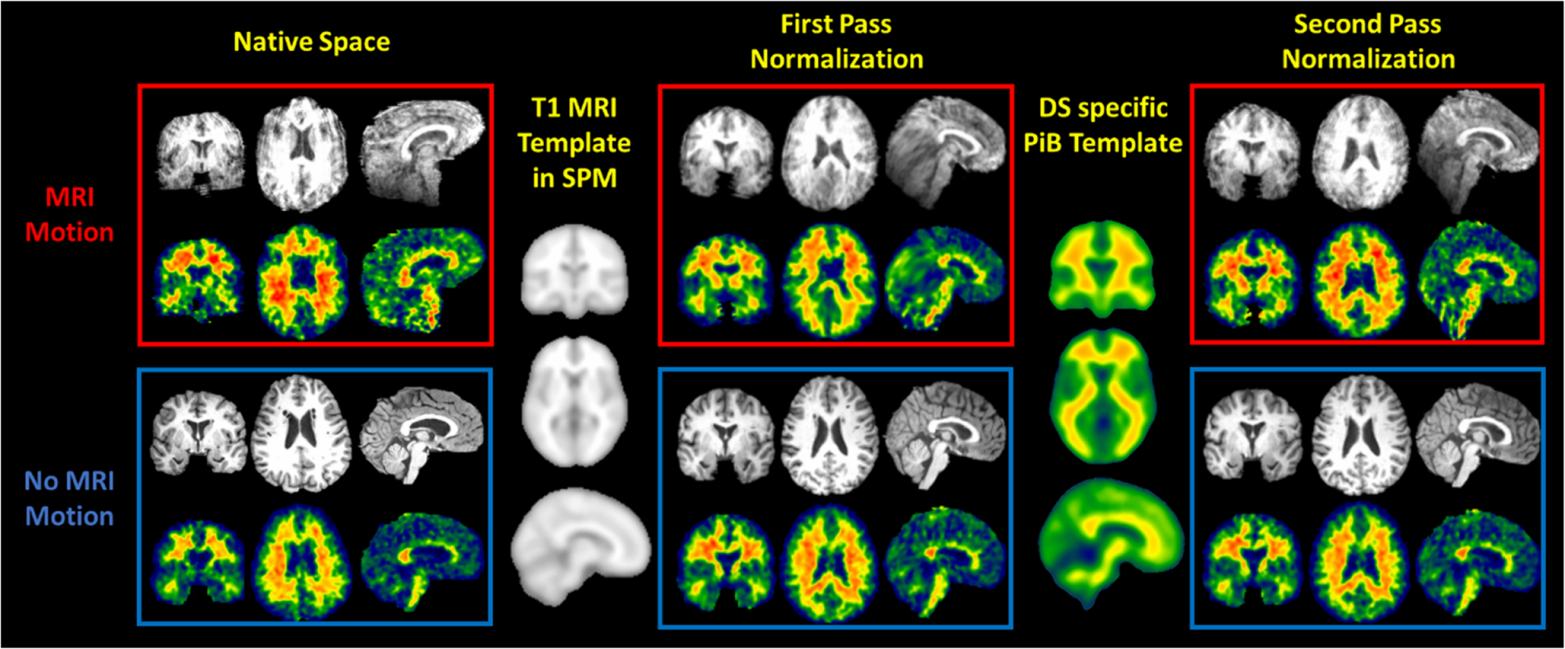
Visual comparison of representative scans with and without MRI motion in native space (red and blue boxes, respectively), and in standardized space after first pass normalization (Native space MRI to T1 MRI template (MNI152)) or second pass normalization (Native space PiB SUVR to DS specific PiB template)
